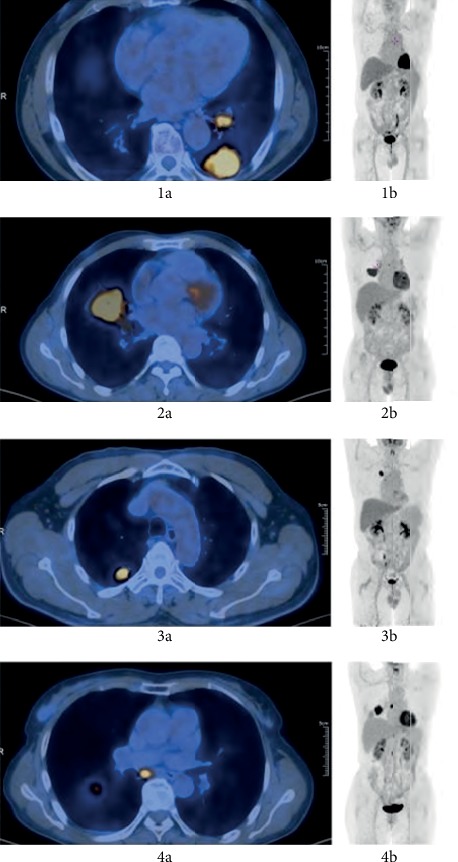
The fusion image (a) and MIP image (b) of patients with pulmonary adenocarcinoma and squamous carcinoma. 1a and 1b: a 66-year-old male patient presented with squamous carcinoma in the inferior lobe of the left lung combined with hilar lymph node metastasis. The SUV_max_ was about 15.1 and Ki67 was about 70, which was presented as non-SN2. 2a and 2b: a 55-year-old male patient showed squamous carcinoma in the middle lobe of the right lung combined with paratracheal lymph nodes. No lymph node metastasis was observed in the right hilum of the lung. The SUV_max_ was about 10.8, and Ki67 was about 60, which was presented as SN2. 3a and 3b: a 67-year-old male patient presented to our hospital due to adenocarcinoma in the superior lobe of the right lung combined with right hilar lymph node metastasis. The long diameter of tumor was about 24.0 mm, and Ki67 was about 30, which was presented as non-SN2. 4a and 4b: a 64-year-old female patient showed adenocarcinoma in the inferior lobe of the right lung combined with lymph node metastasis beneath the eminence. There were no lymph node metastases in the right hilum of the lung. The long diameter of the tumor was about 47.0 mm, and Ki67 was about 70, which was presented as SN2.
